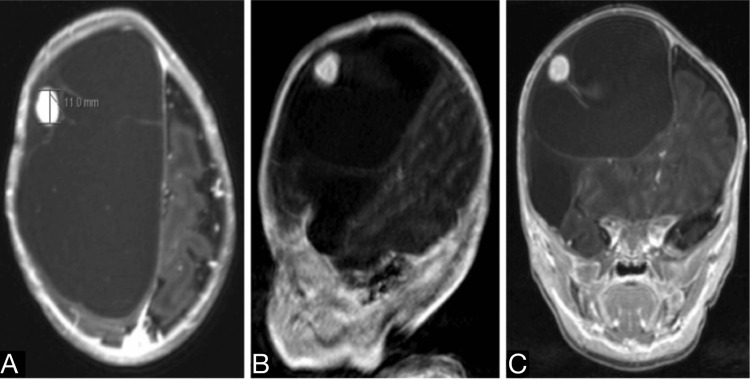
(A) Magnetization prepared rapid acquisition gradient echoes (MPRAGE) axial T1-weighted contrast-enhanced MRI, (B) sagittal T1-weighted contrast-enhanced MRI, (C) coronal T1-weighted contrast-enhanced MRI demonstrate a well-defined oval-shaped lesion that is homogeneously enhancing located at the lateral aspect of the mass.
